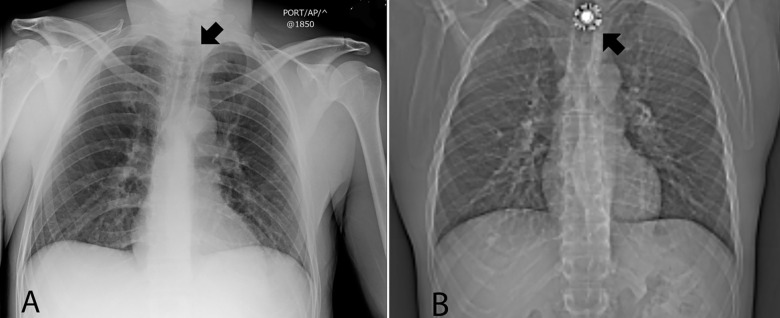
A. Normal initial chest radiograph absent of any ingested sprinkler head (arrow). B. Computed tomography scout view of chest now demonstrating the ingested sprinkler head (arrow).
